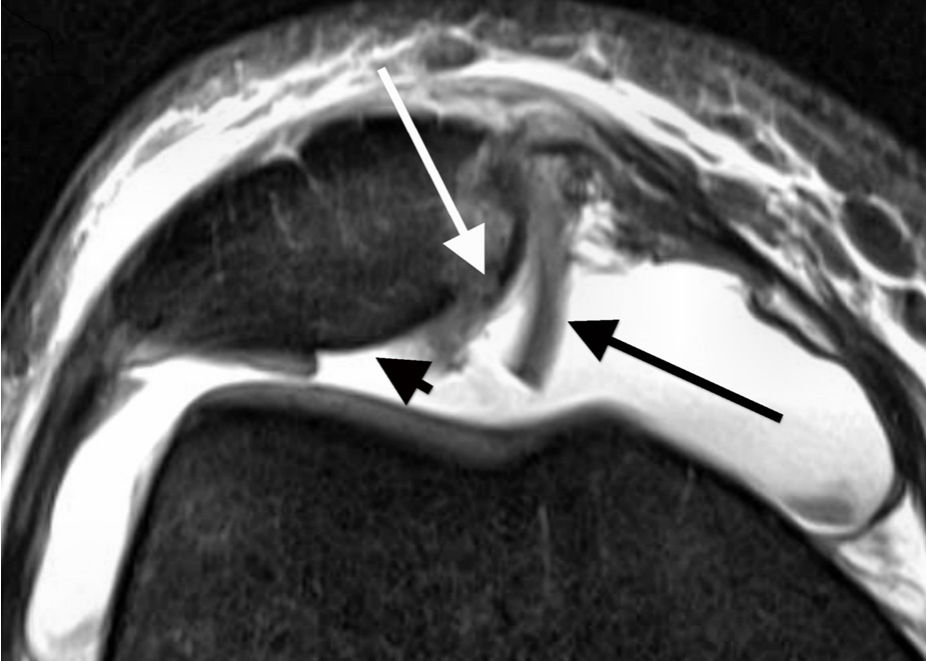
Axial PD-weighted TSE MRI of a 41-year-old female with a first LPD. At the medial facet of the patella, a full-thickness defect of the cartilage (grade 4) with fracture of the subchondral bone (long white arrow) and a free cartilage fragment (long black arrow) is visible. Furthermore, a full thickness cartilage defect at the central dome of the patella (short black arrows) is depicted. MR imaging of chondral or osteochondral fragments, cortical steps or bone destructions provides additional information when planning surgery, such as a refixation.
